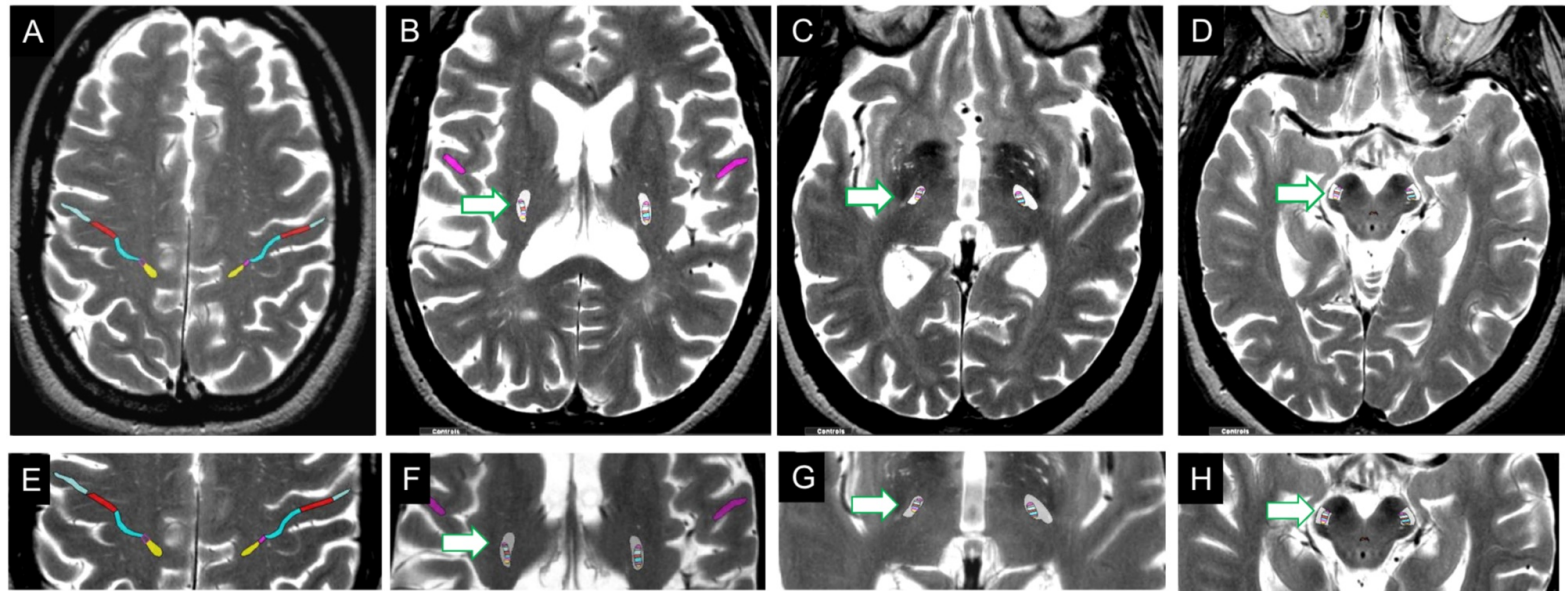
Functional-anatomical localization using Anatom-e The primary motor cortex (A, F) and corticospinal tracts (B-D, F-H) are shown on a reference T2-weighted MRI. The somatotopic organization of each is indicated by different colors (yellow-leg; purple-trunk or shoulder; light blue-shoulder/arm; red/lighter blue- forehead and face). The CTS is outlined in white (indicated by the white arrow with a green outline) at the levels of the centrum semiovale (B,F), basal ganglia (C,G), and midbrain peduncle (D,H). CST: corticospinal tract
